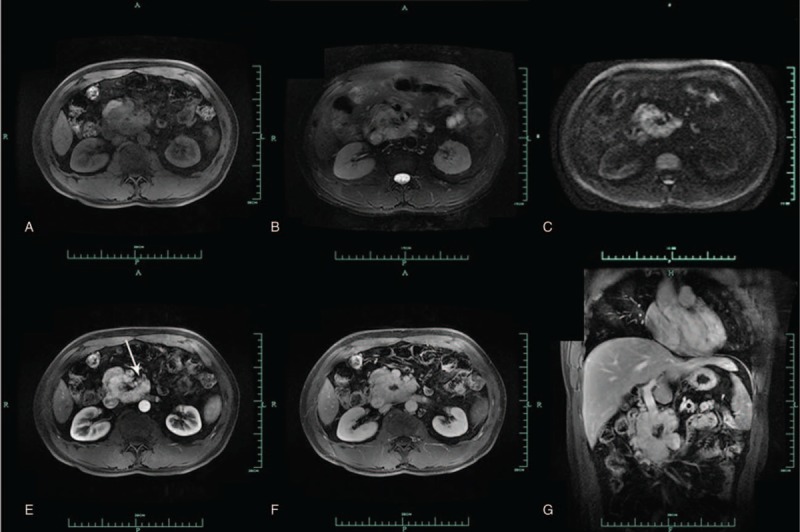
(A) Routine pancreatic MRI showing slightly heterogeneous hypointensity of the pancreatic head mass on T1WI, with a clear boundary. (B) T2WI of the mass, showing heterogeneous, slight hyperintensity. (C) DWI sequence of the mass, showing slight hyperintensity. (D) Contrast-enhanced pancreatic MRI showing severe mass enhancement, with no enhancement of the central focus. Draining vein of the mass in the arterial phase are also observed peripherally (arrow). (E) Slightly decreased venous phase enhancement of the mass, which still shows relative hyperintensity. (F) Coronal enhancement of the mass, tending to be homogeneous and hyperintense, with no central enhancement, and occurring in the delayed phase.
